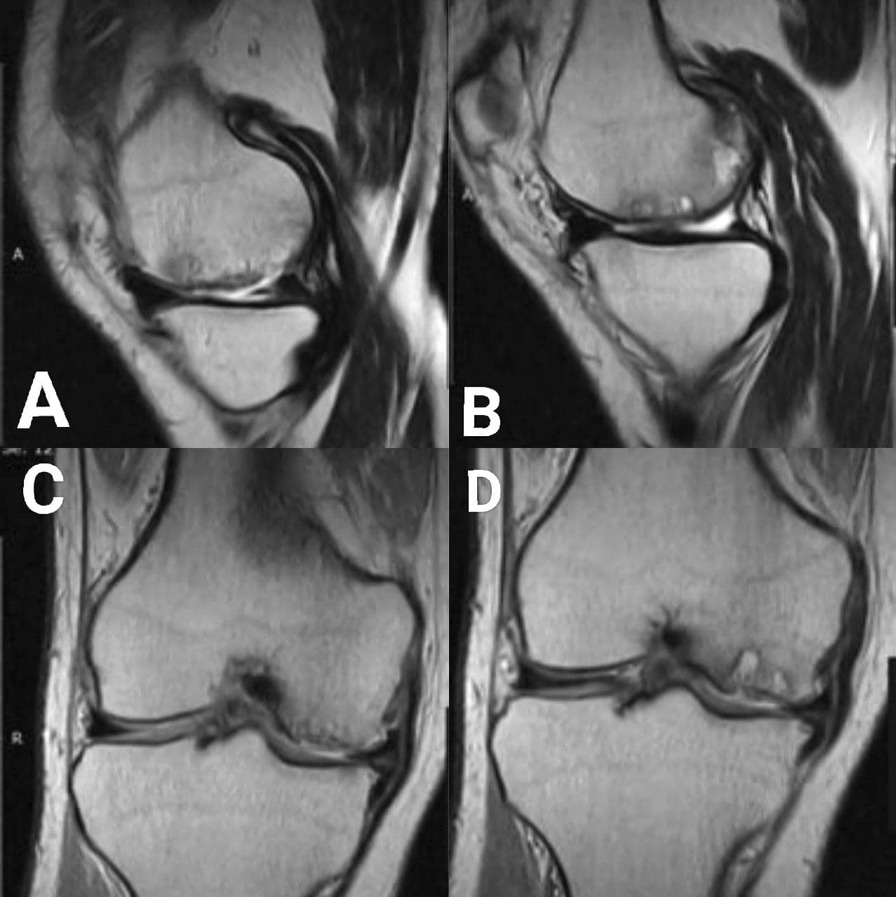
A–D: A, B T2 sequence sagittal image showing the adherence of the delaminated flap in most parts (MRI center did not provide sagittal images in PDW sequence). C, D PDW sequence coronal image showing the irregular subchondral bone and the adherence of the many parts of the delaminated flap with loss of some chondral parts
